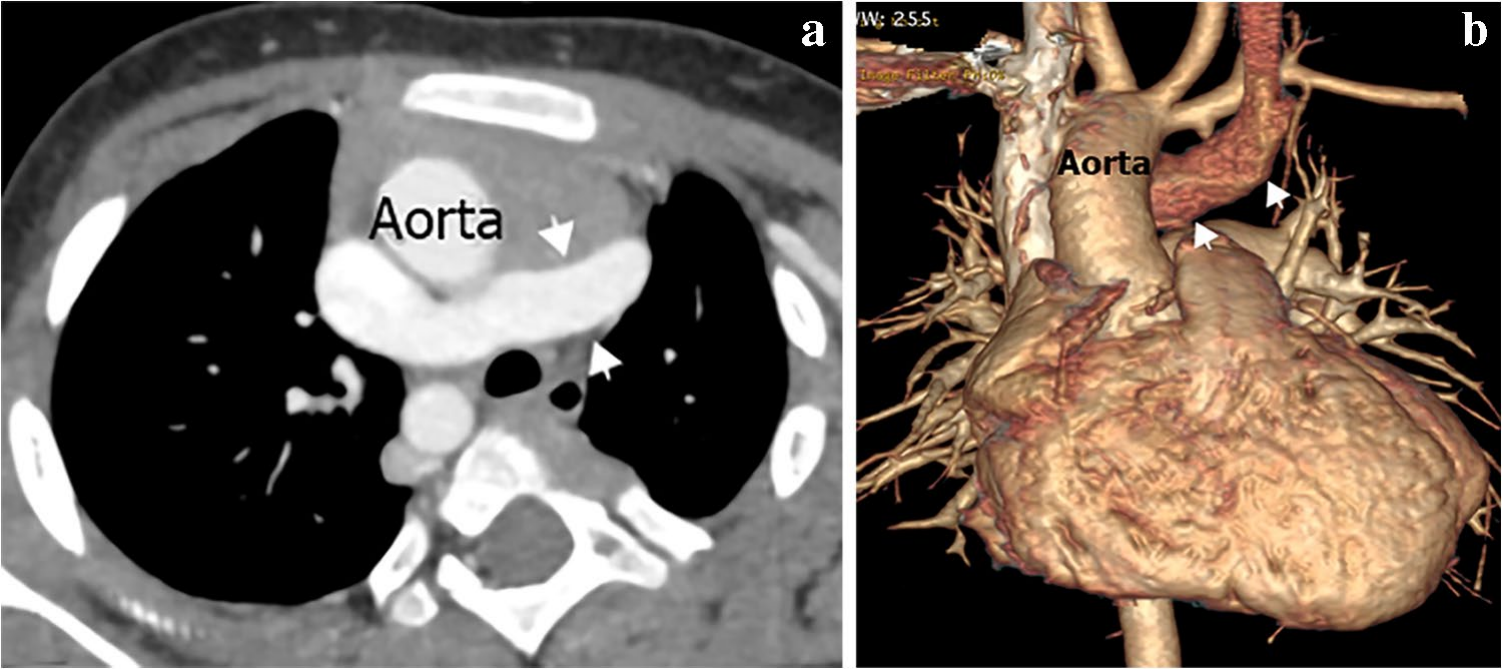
A retroaortic brachiocephalic vein with no other anomalies in a 3-year-old boy. a, b Axial (a) and volume-rendered (b) computed tomography images show retroaortic brachiocephalic vein (*arrows*)
